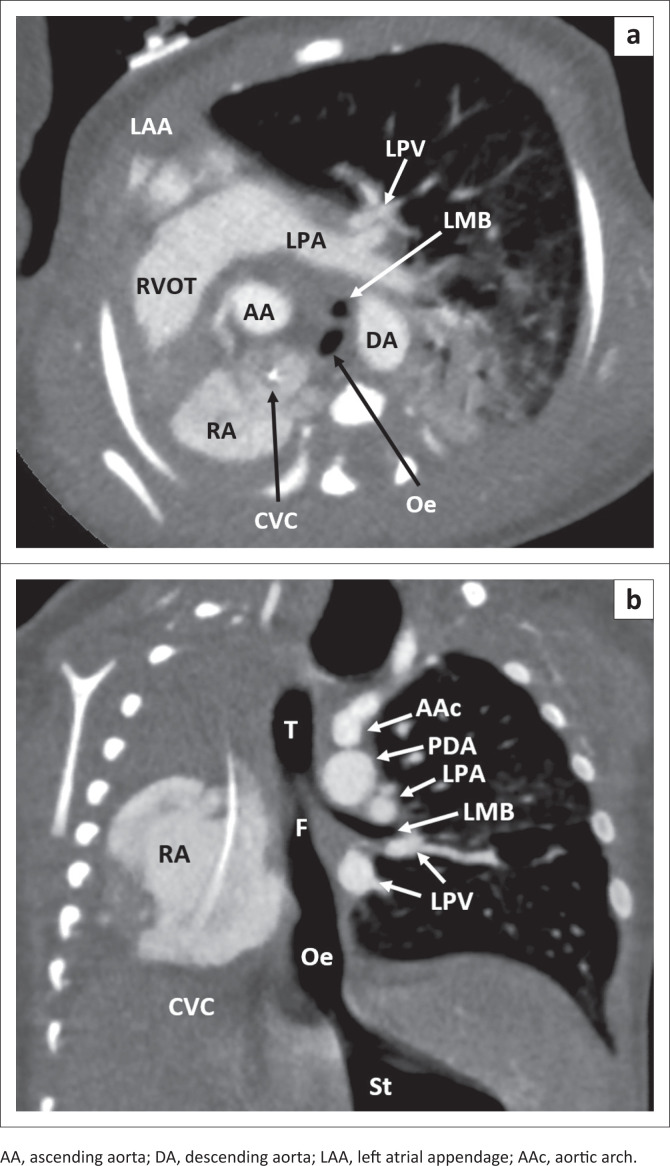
Computed tomography angiography thorax in the axial (A) and oblique coronal (B) planes reveals absence of the right lung, bronchus and pulmonary vessels with ipsilateral mediastinal shift. The left pulmonary artery (LPA) and veins (LPV) are present with a hyperinflated left lung. The trachea (T) divides into the left main bronchus (LMB) and communicates with the lower oesophagus (Oe) via a fistula (F) consistent with a tracheo-oesophageal fistula. The patent ductus arteriosus (PDA) is large. The tip of the central venous catheter (CVC) is in the right atrium (RA).
